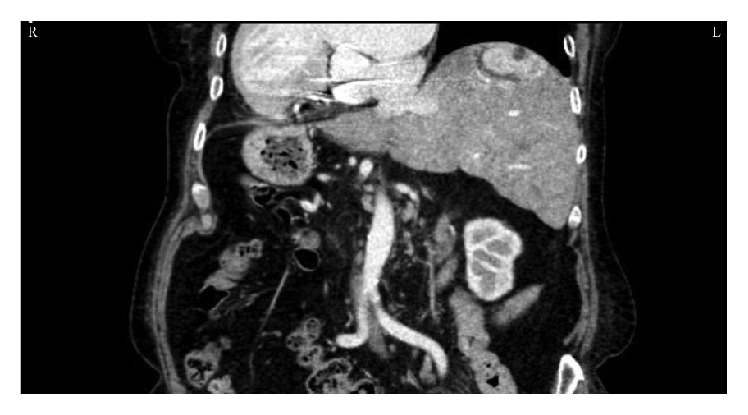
Coronal CT images of angiomyolipoma in a patient with situs inversus totalis.
